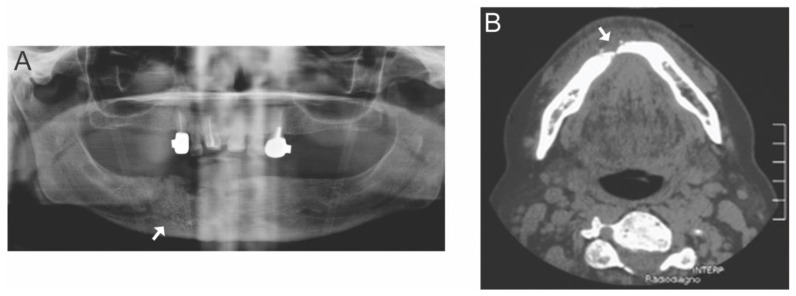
Dual diagnostic radiology of osteoradionecrosis. Right mandibular osteoradionecrosis was diagnosed with a combination of panoramic radiograph ((A), white arrow) and axial computerized tomography soft tissue window ((B), white arrow) at the level of mandibular body. (Images kindly provided by Dr. Mel Mupparapu, from the University of Pennsylvania, in Philadelphia, PA, USA).
